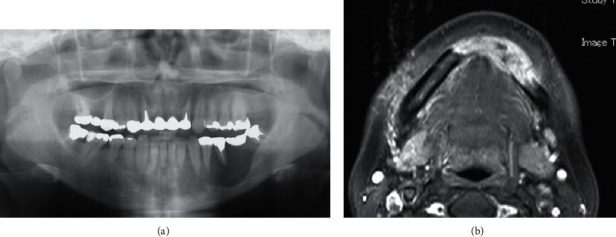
(a) Panoramic radiograph revealing a posterior well-demarcated radiolucent area and anterior irregular area. (b) Magnetic resonance images (T1 weighted) showing a tumor in the midline to the left side of the mandible with a high-signal mass measuring 42 × 13 mm.
